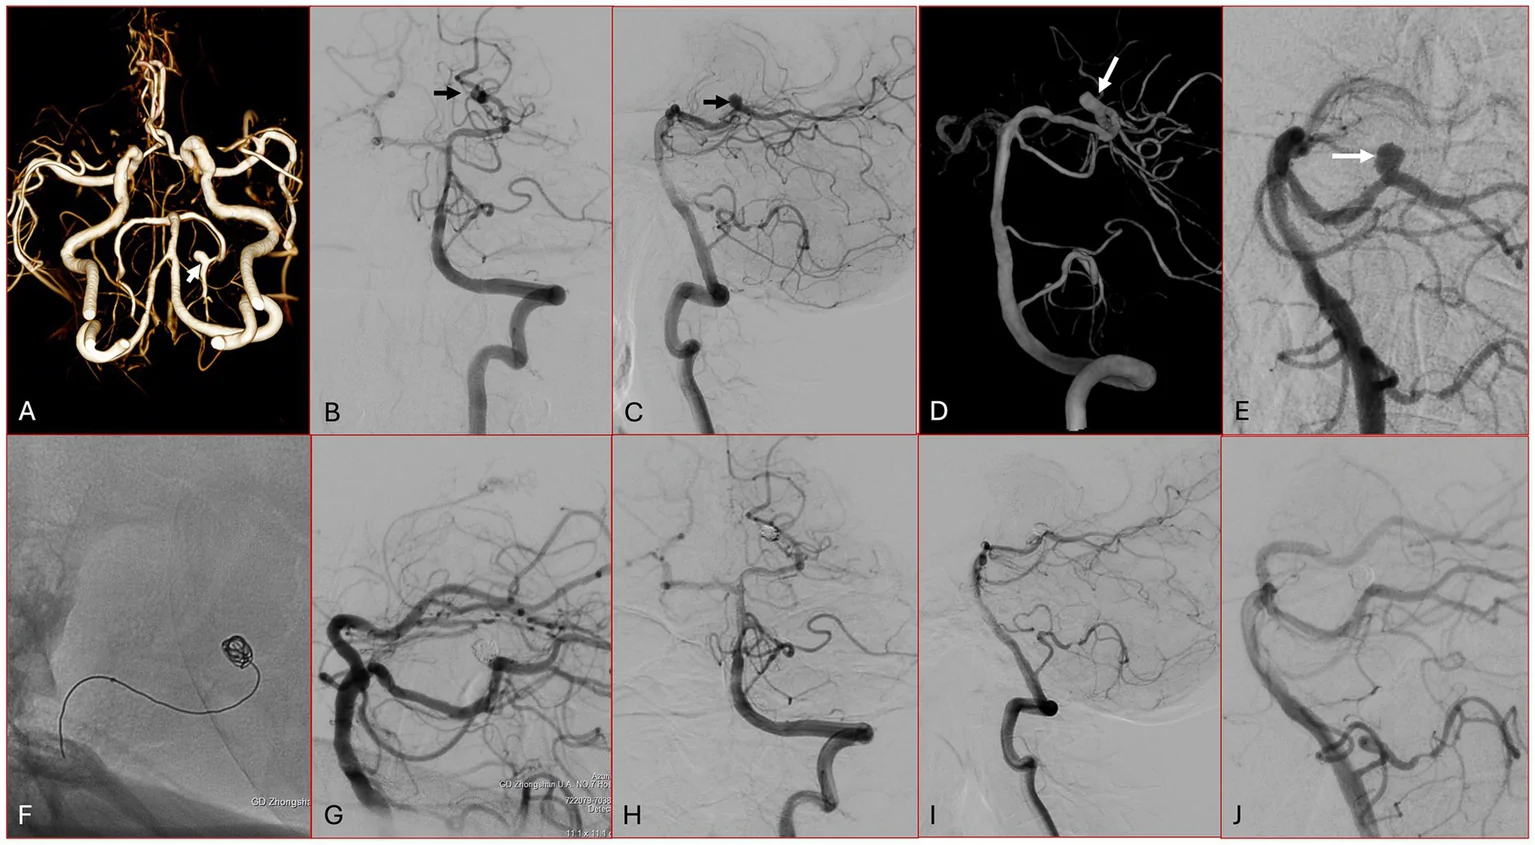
Case 4. 69-year-old asymptomatic male patient with an incidentally detected aneurysm. (A) Head CTA demonstrates a 5 × 4 mm saccular aneurysm (white arrow) with a 2.5-mm neck, located at the P2P segment of the left posterior cerebral artery (PCA). (B,C) Left vertebral artery angiograms [anteroposterior (B) and lateral (C)] demonstrate a saccular aneurysm (black arrow) at the P2P segment of the left PCA. (D,E) Volume-rendered (VR) reconstruction of 3D-DSA (D) and working view of left vertebral artery angiogram (E) demonstrate a saccular aneurysm (white arrow) at the P2P segment of the left posterior cerebral artery (PCA). (F) X-ray spot film demonstrates coil embolization of the aneurysm following 3D framing coil deployment. (G) Immediate post-procedural left vertebral artery angiogram shows complete occlusion of the aneurysm. (H–J) Follow-up angiograms at 12 months post-procedure [anteroposterior (H), lateral (I), and tangential (J)] demonstrate stable complete aneurysm occlusion.
